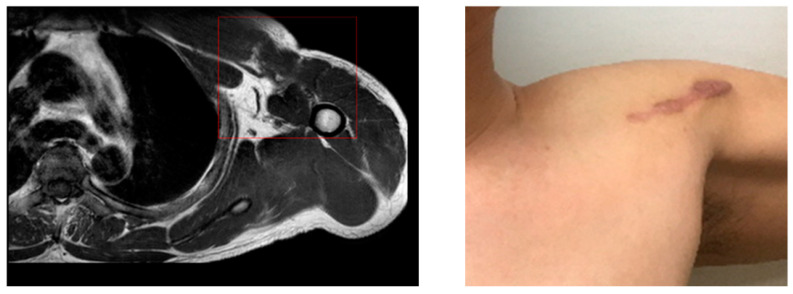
Patient’s MRI and injury site.
